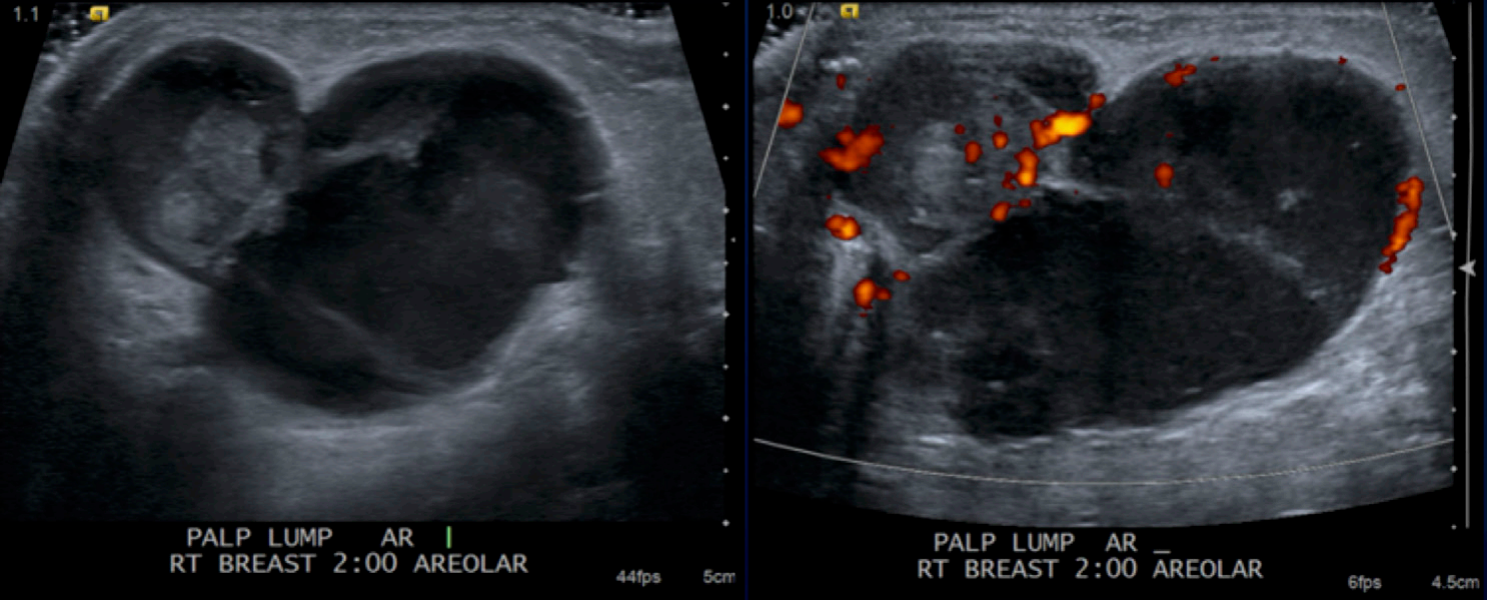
Ultrasound images in the right breast at 2:00, subareolar demonstrates a
